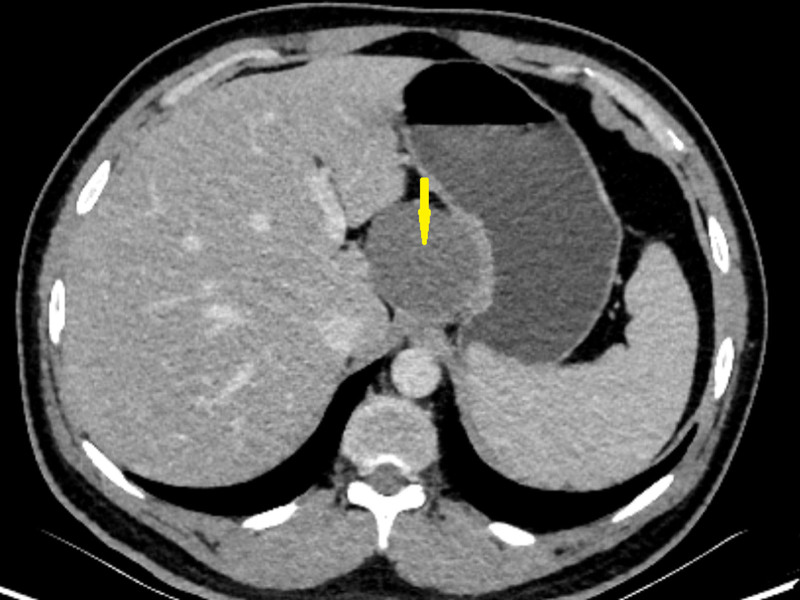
Enhanced CT. A mass-like slightly low-density shadow in the hepatogastric hiatus, about 5.8cm × 4.7cm in size, mild homogeneous enhancement of the lesion on enhanced scan, suspected mesenchymal stromal tumor. The yellow arrow points to this tumor. CT = computed tomography.
